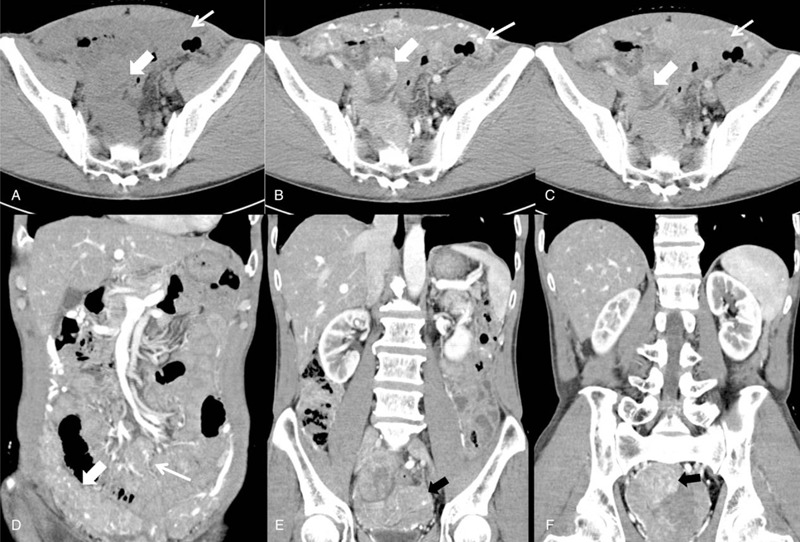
A: Axial view plain CT scan showed a large soft tissue mass involving the pelvic peritoneum (thick arrow) and greater omentum (thin arrow); B: contrast-enhanced CT scan showed significant enhancement during arterial phase (thick arrow) and dilated feeding artery supporting the omental lesion (thin arrow); C: non-enhanced necrotic area (thick arrow) could be seen within the pelvic lesion during parenchymal phase, and the omental lesion demonstrated a homogeneous enhancement; D-F: coronal view of greater omental (thick arrow), mesentery (thin arrow) and pelvic (black arrow) lesions.
